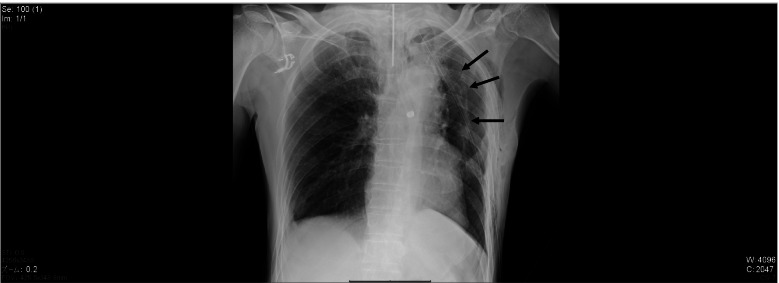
Postoperative chest X-ray image. The volume of the left lung was reduced. The allows indicate the visceral pleura
